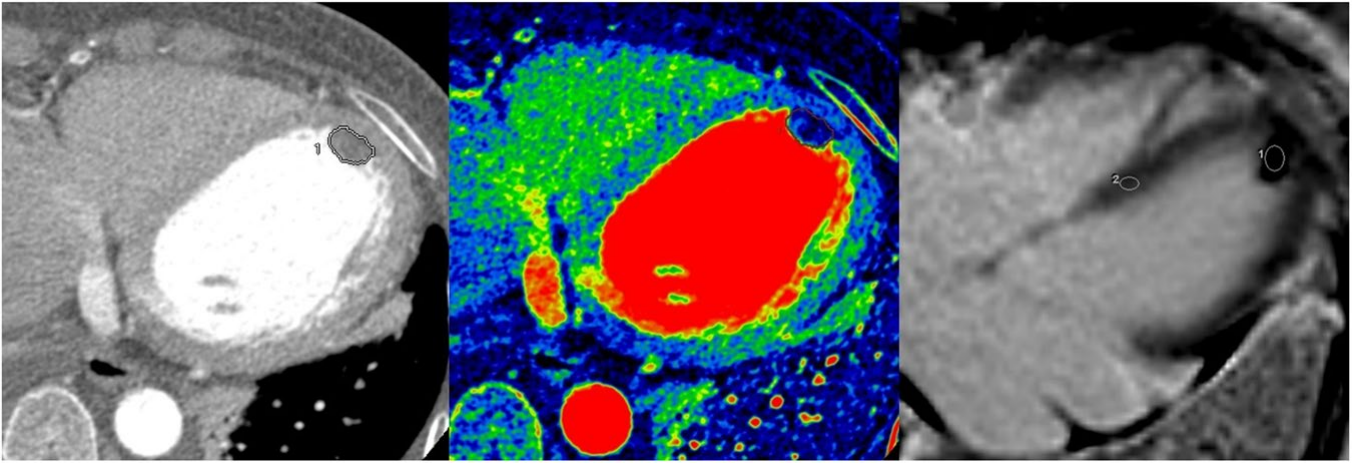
A 53-year-old female patient diagnosed with a thrombus. (A) On enhanced CT, a 1.5 × 1.0-cm well-defined mass was noted in the apex of left ventricle. The volume of the mass was 0.81 cm^3^ and the CT attenuation value of the intra-cardiac mass was 59.0 HU. (B) On the iodine (water) image, the iodine concentration within the VOI was 1.05 mg/ml. (C) On LGE image, the mass shows dark signal intensity without enhancement; the SI ratio of the mass was 0.89.
